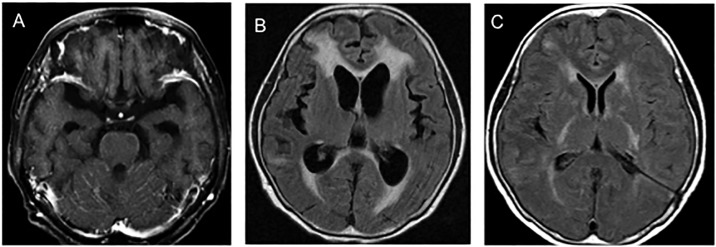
MR image before and after shunt surgery. (A) MR image shows linear enhancement along the cerebellar folia. (B) FLAIR image obtained prior to VP shunt shows enlarged lateral ventricles with periventricular high intensity. (C) FLAIR image after VP shunt shows decreased size of the lateral ventricles and improved periventricular high intensity three months after shunting.
